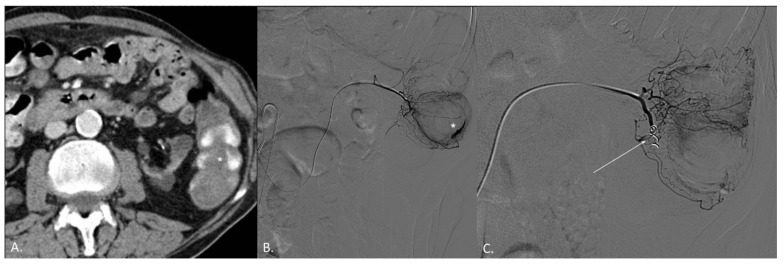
(A–C) CT angiography (CTA) and digital subtraction angiography (DSA) of an 82-year-old female patient. (A) CTA demonstrates active endoluminal bleeding (*) from left colon angiodysplasia. (B) DSA obtained with catheterization of the left colic artery (LCA) confirms CTA findings. (C) DSA obtained with catheterization of the LCA after coil embolization. No active bleeding evidence.
